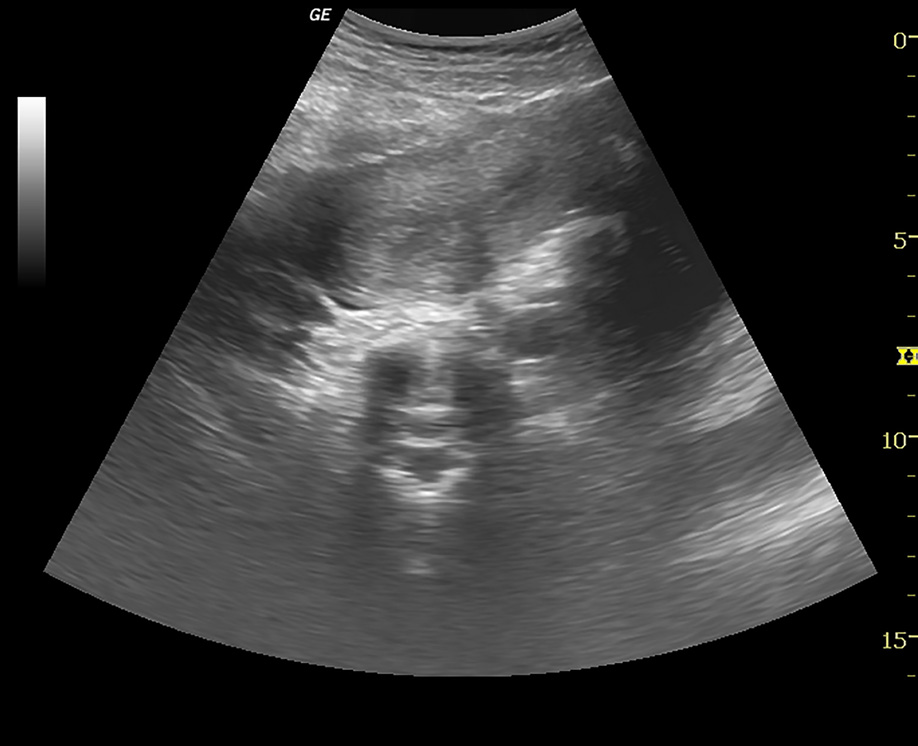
Transverse ultrasound image showing two uterine cavities
